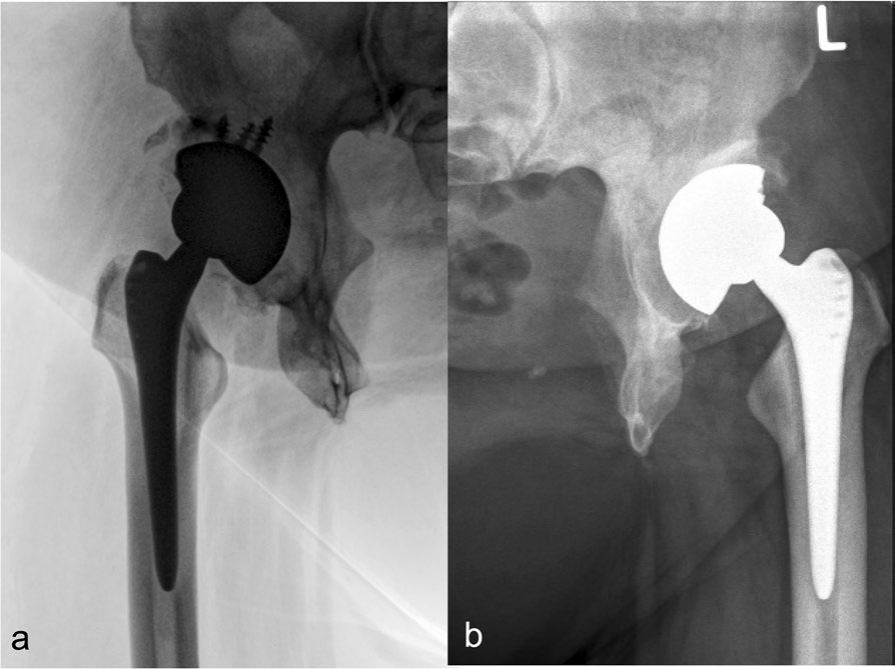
a) Postoperative radiography of the right hip with the osteoconductive cup (TM modular cup) size 56 mm with an additional anteverted liner 20° combined with distally fixed stem (Alloclassic-SL); b) Postoperative radiography on the left hip at 3 months
